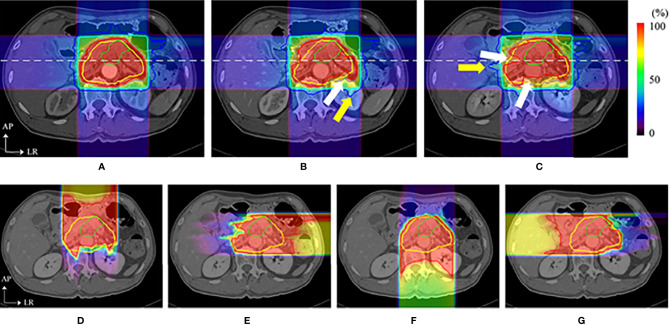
Dose distributions of a carbon ion RT in axial sections. The top images show the dose distribution of planning CT (A), the dose distribution with a delay time of (B) 35 s (intra-CT), and (C) 145 s (intra-CT), respectively. Beam overshoot (yellow arrows) and undershoot (white arrows) were observed at scan intervals of 35 and 145 s. The bottom images show the dose distributions of (D) 0°, (E) 90°, (F) 180°, and (G) 270° at 145 s. The green and yellow lines show the shapes of the gross tumor volume and the clinical target volume, respectively. The rainbow contours show the dose distribution (35) (Source: Elsevier. Used with permission).
